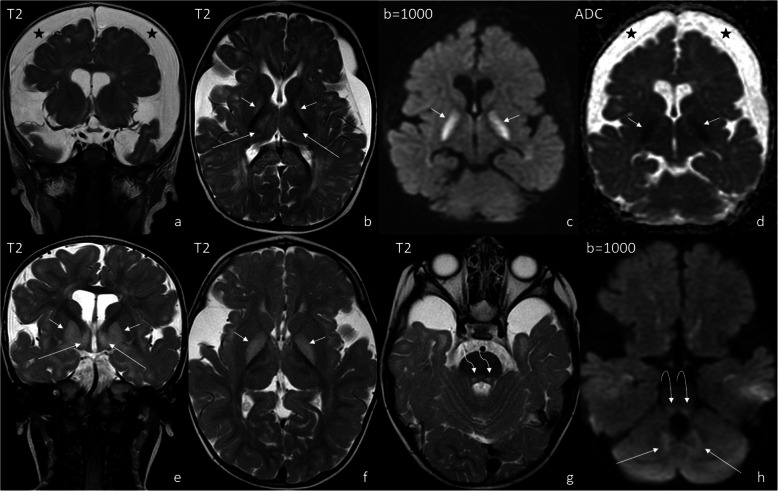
Typical MRI features of GA-1. Scan (1) at the age of 8 months - upper row, scan (2) at the age of 21 months – lower row. Abnormal T2-hyperintensity and diffusion restriction of the globi pallidi in both examinations (b-d and e,f, short arrows). Dentate nuclei (h) and thalami (b,e) with T2-hyperintensity and diffusion restriction in both examinations (long arrows). Medial lemnisci in dorsal pons with T2-hyperintensity and diffusion restriction in both examinations (g,h, curved arrows). Symmetrical subdural hygromas (asterisks) detected on scan (1) (a-d) regressed in scan (2) (e-h)
